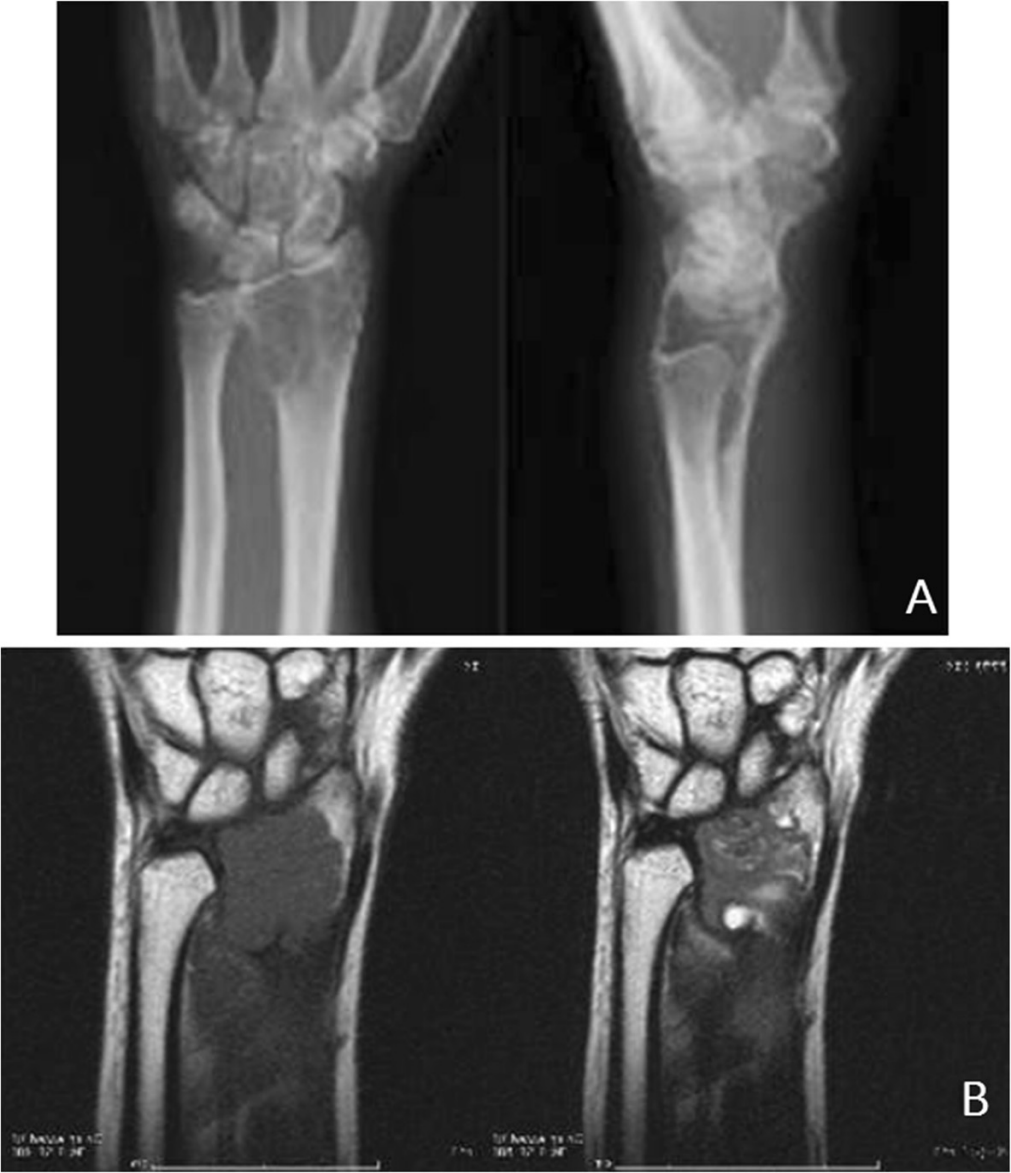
The preoperative X-ray (A) and magnetic resonance imaging (B) at the first visit.
